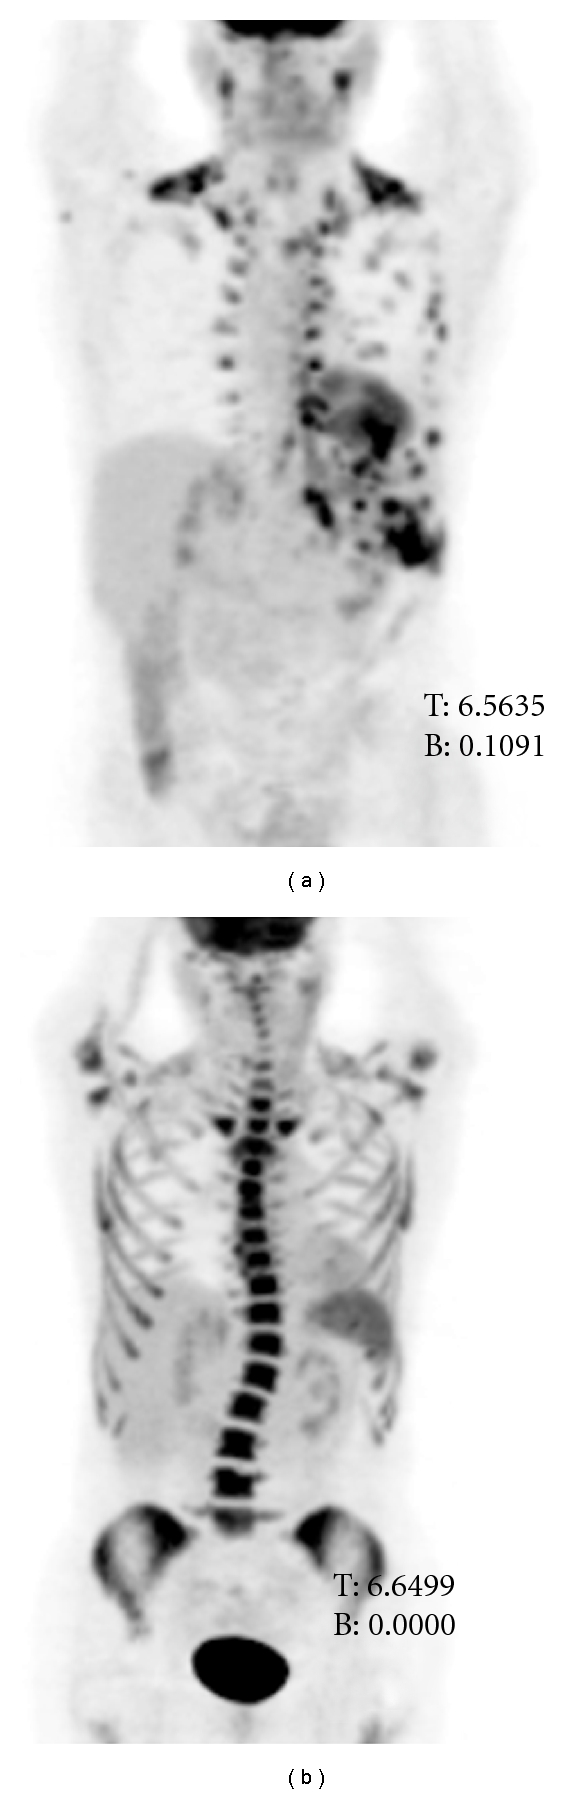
(a) The pretherapy anterior (0° degree) maximum intensity projection image of the PET/CT study of the patient demonstrating multiple pleural-based nodular metastatic lesions in the left chest along with prominent FDG uptake also seen in the activated brown fat tissue in multiple locations. (b) The six-months posttherapy anterior (0° degree) maximum intensity projection image of the PET/CT study of the patient showing complete resolution of the previously seen FDG avid lesions associated with intense bone marrow FDG activity and increased splenic activity seen due to therapy-induced bone marrow stimulation.
